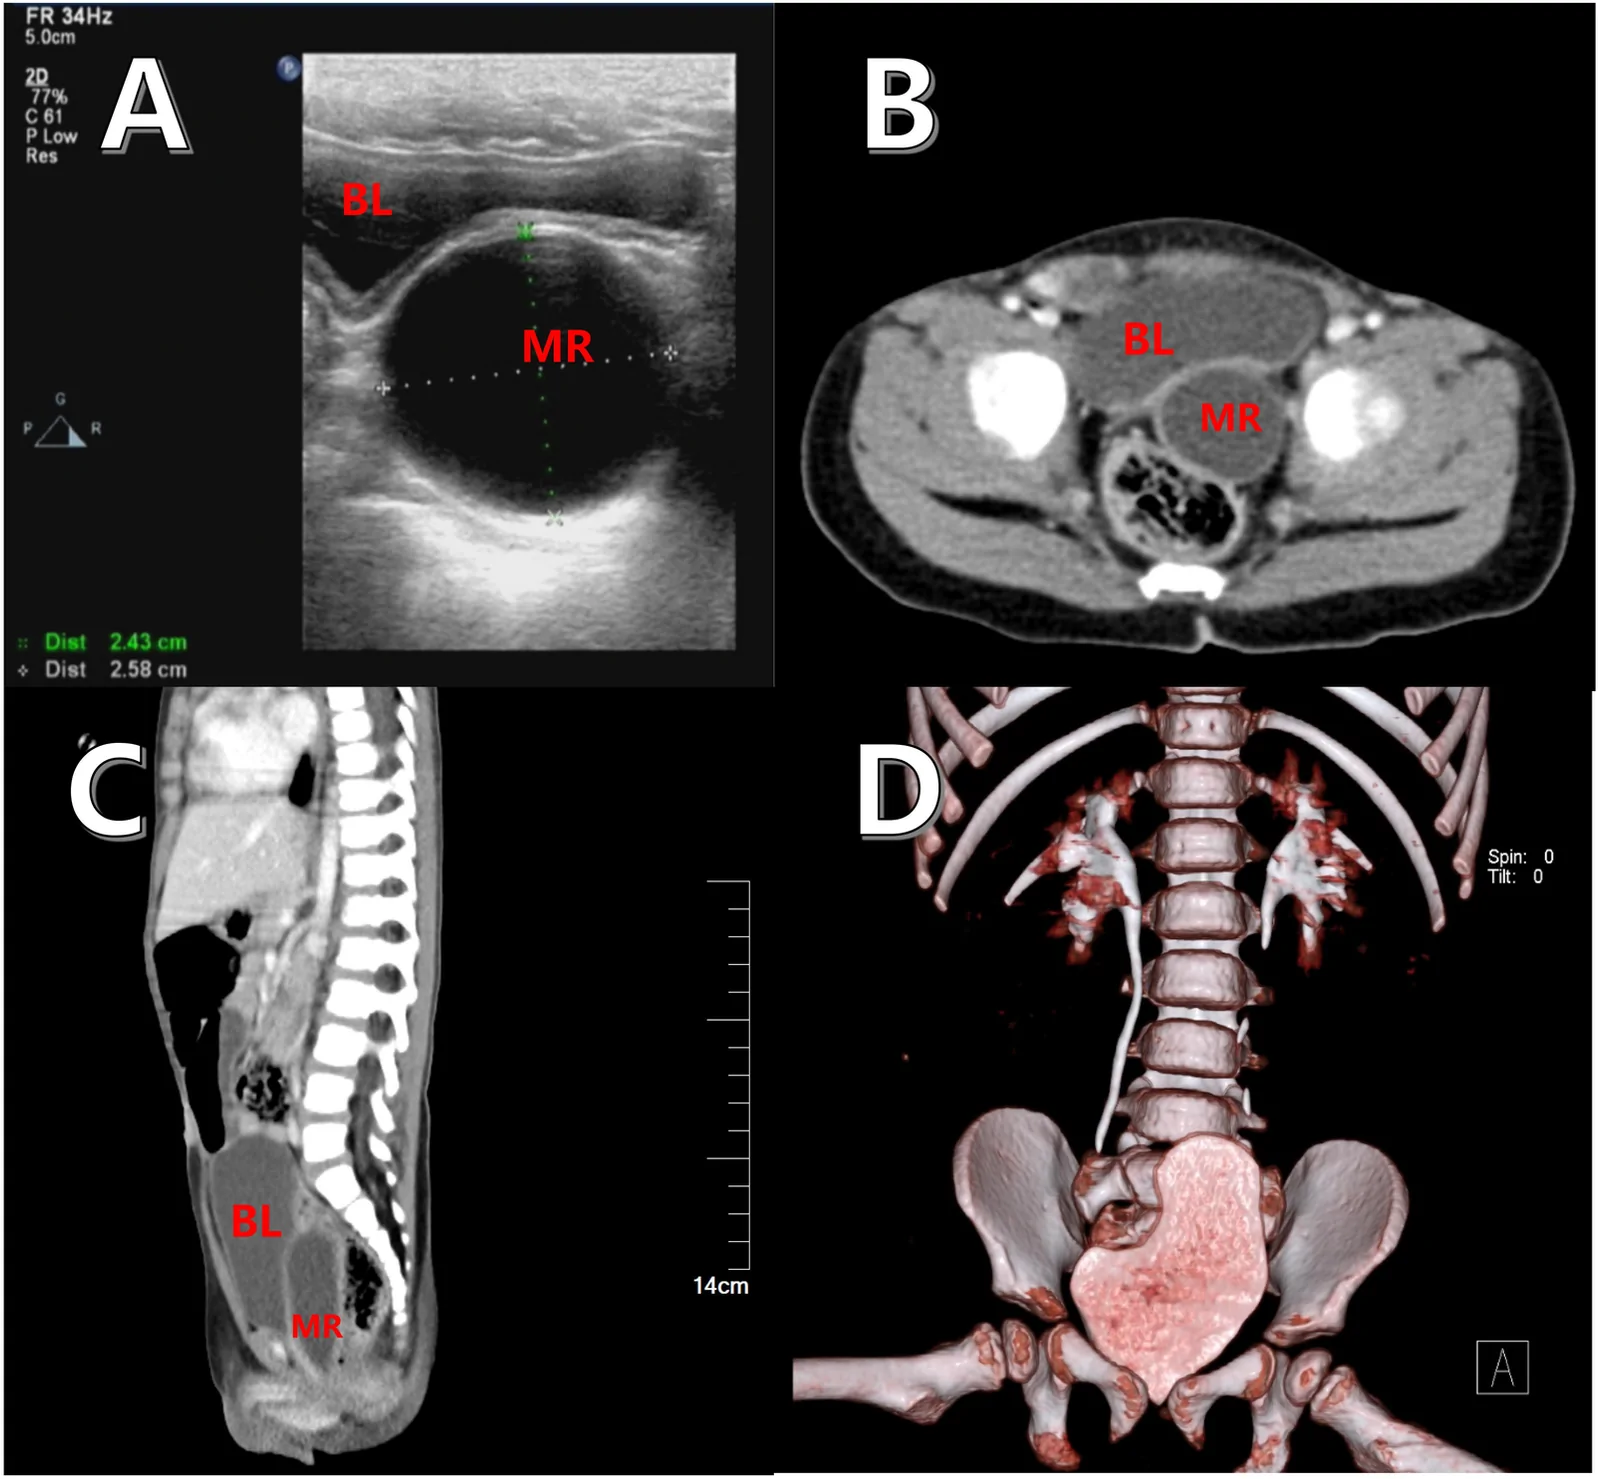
Preoperative imaging of the retrovesical cystic lesion subsequently confirmed as a Müllerian remnant complex. (A) Transabdominal ultrasonography shows a well-circumscribed anechoic retrovesical cystic lesion (MR) immediately posterior to the bladder (BL); calipers indicate orthogonal diameters of 2.43 and 2.58 cm. (B) Axial contrast-enhanced computed tomography (CT) demonstrates a midline retrovesical cystic lesion (MR) posterior to the bladder (BL). (C) Sagittal CT reconstruction shows the lesion (MR) in the midline, posterior and caudal to the bladder (BL), with distal tapering toward the perineum. (D) Three-dimensional volume-rendered CT reconstruction of the urinary tract shows no upper urinary tract dilation. BL, bladder; CT, computed tomography; MR, Müllerian remnant.
